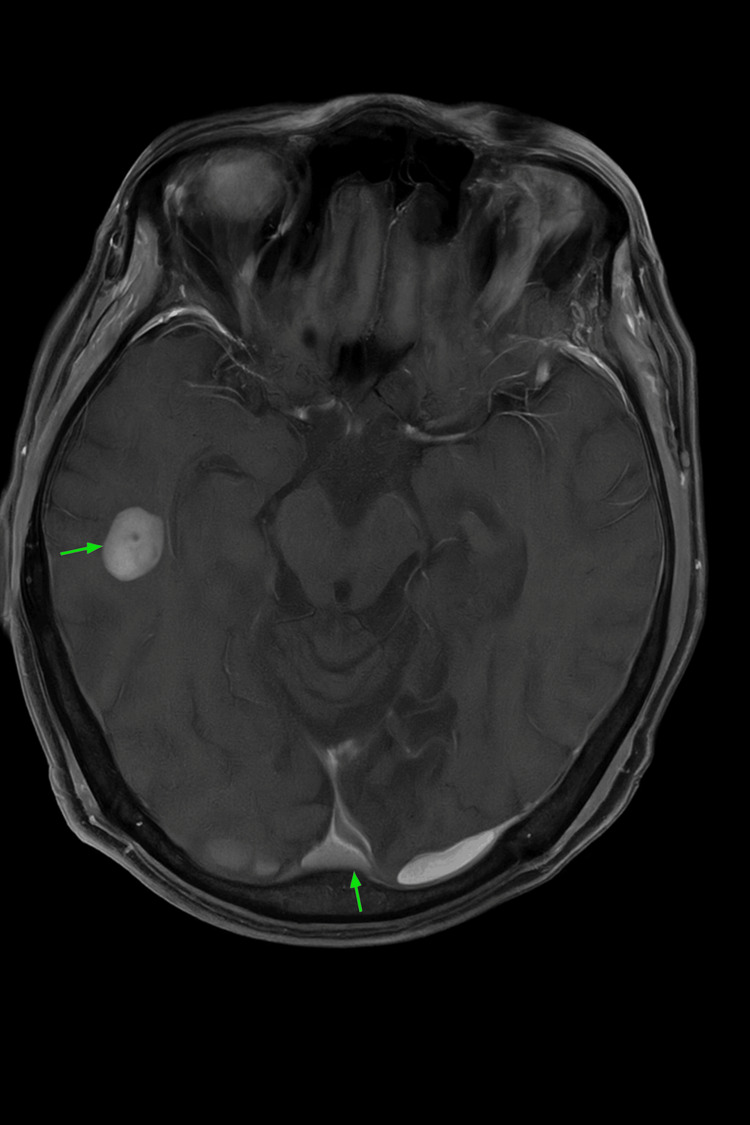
MRI of the brain showing enhancement in the bilateral supratentorial white matter and intracranial metastases without mass effect
